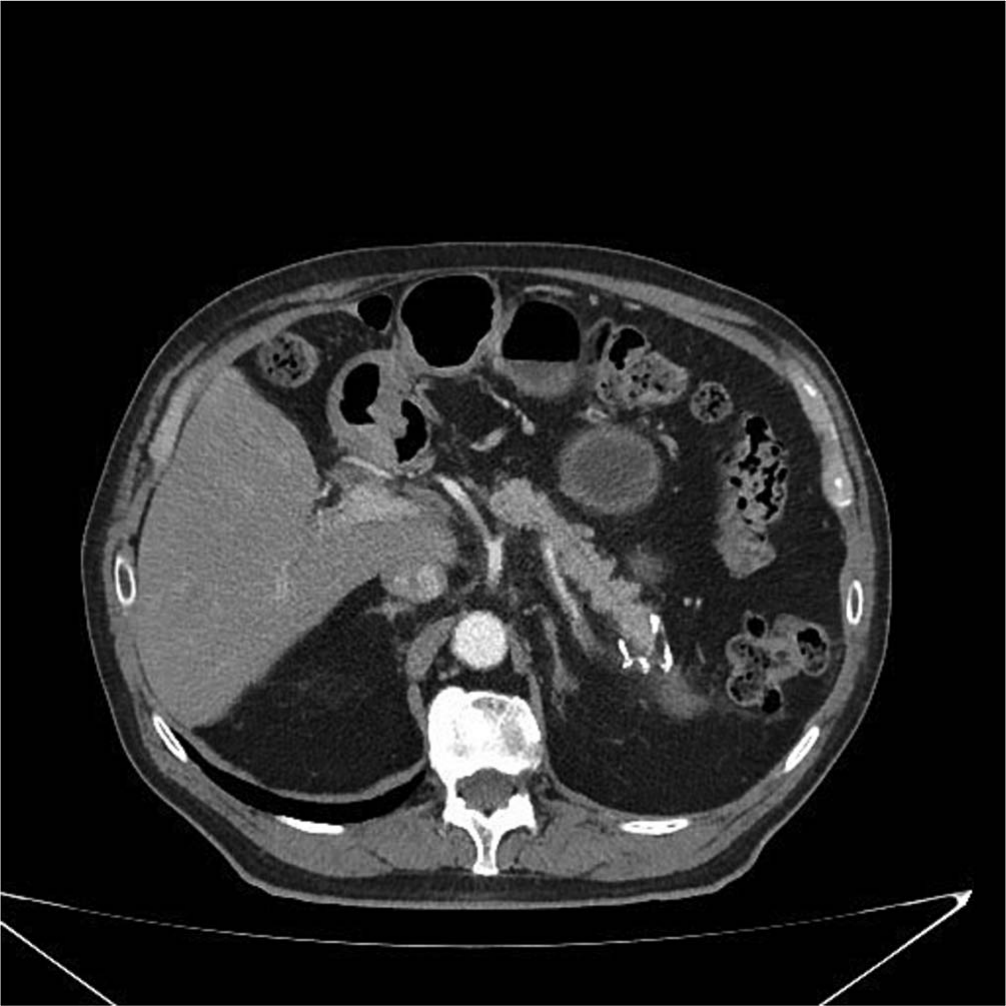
Postoperative CT scan showing surgical clips in the pancreatic tail region and a hypodense nodular lesion with lipomatous characteristics, measuring 2.1 cm, adjacent to the surgical site. No evidence of recurrence or complications is observed.
